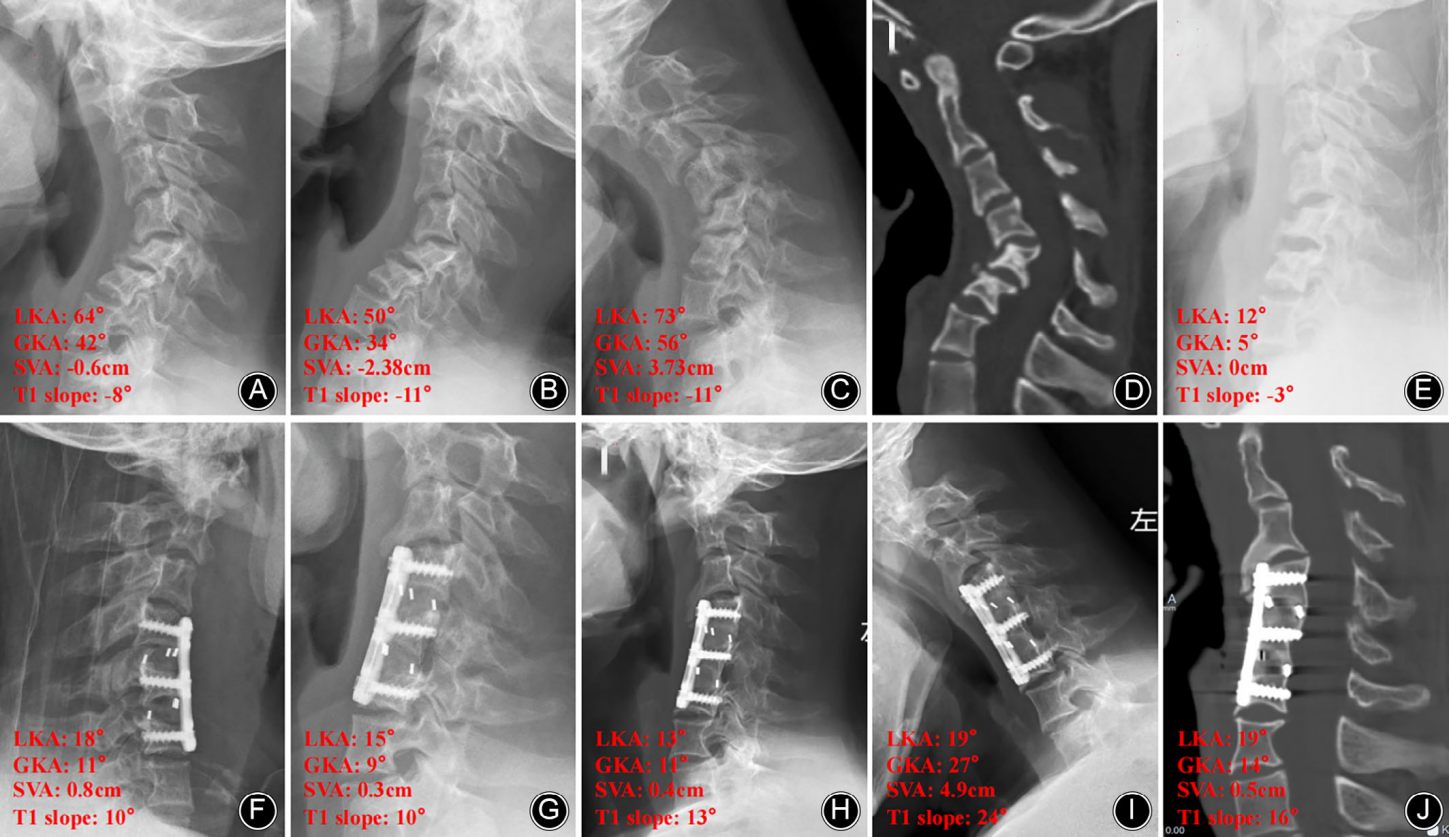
Case 7. A 16‐year‐old boy with NF‐1 cervical kyphosis. Preoperative lateral X‐ray (A) and computed tomography (CT) scan (D) showing significant scallop of C5 and C6 vertebrae. The local kyphosis angle (LKA) and global kyphosis angle (GKA) were 64° and 42°, respectively. Preoperative flexion–extension X‐ray (B, C) showing fixed cervical kyphosis. The LKA and GKA were 50° and 34°, respectively, in extension X‐ray, and 73° and 56°, respectively in flexion X‐ray (C). Post‐traction X‐ray (E) showed that the LKA and GKA were 12° and 5° after 10 days of continuous skull traction. Postoperative X‐ray (F) showed that the LKA and GKA were 18°and 11°after C4–6 anterior cervical corpectomy and fusion. Kyphosis correction was well maintained based on postoperative 3 months X‐ray (F), 8 months X‐ray (G), 57 months flexion X‐ray (H), and 57 months extension X‐ray (I), without instrumentation failure (J). At 57‐month follow‐up, the postoperative LKA and GKA reduced to 19° and 14°, respectively.
